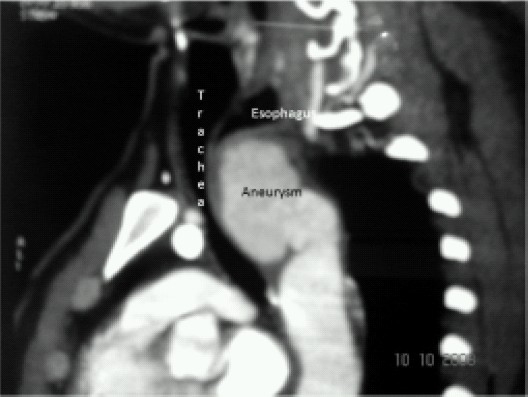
Computed tomographic scan showing compressed trachea and esophagus
